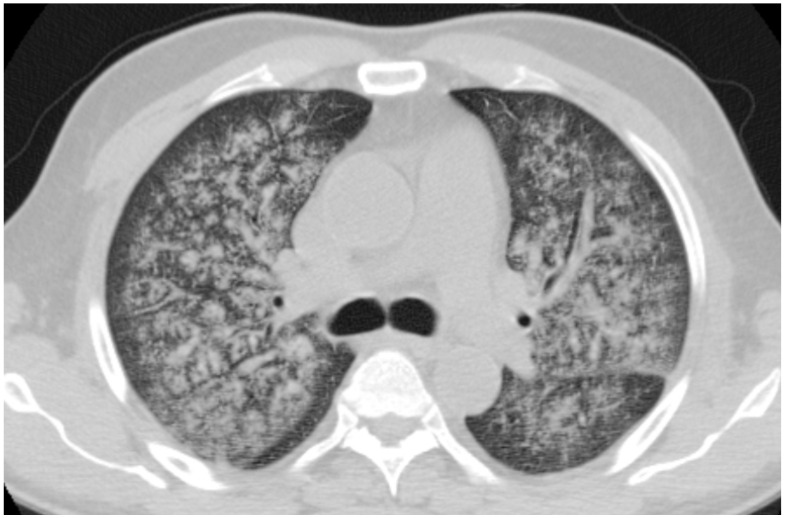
A 64-year-old, male, with fever, weakness, hemoptysis and hematuria. Diagnosis of granulomatosis with polyangiitis. Axial CT shows diffuse hemorrhagic alveolitis diffuse alveolar hemorrhage.
